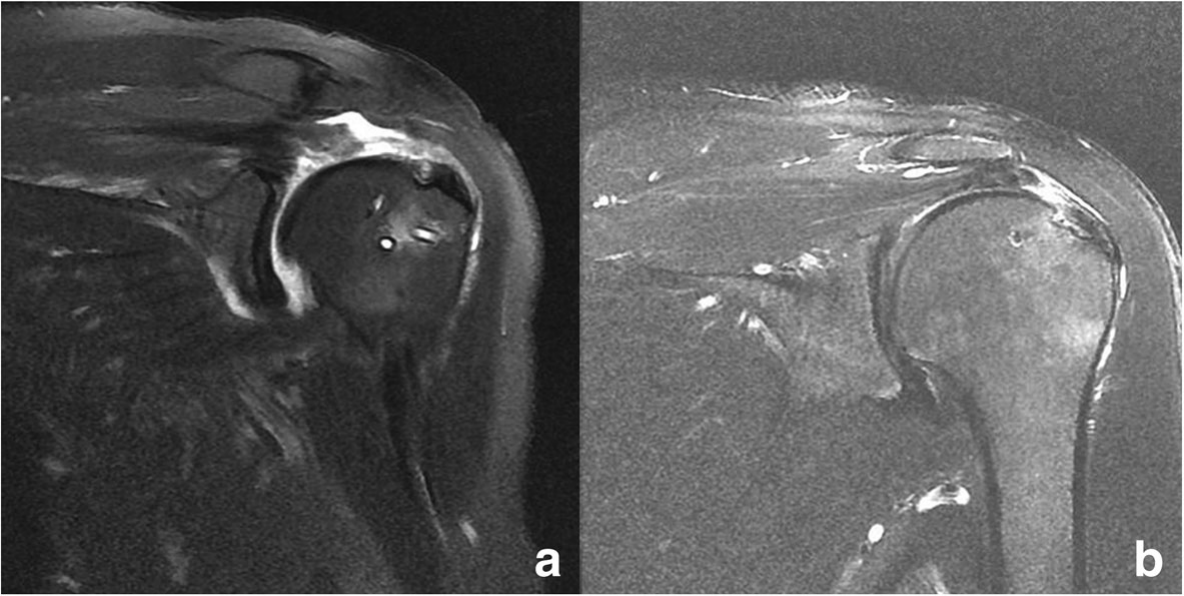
Follow-up T2-weighted magnetic resonance imaging at 6 months post-operation shows medially ruptured tendons and a healed footprint of a repaired rotator cuff (type II re-tear). a Conventional suture-bridge technique. b Knotless suture-bridge technique
